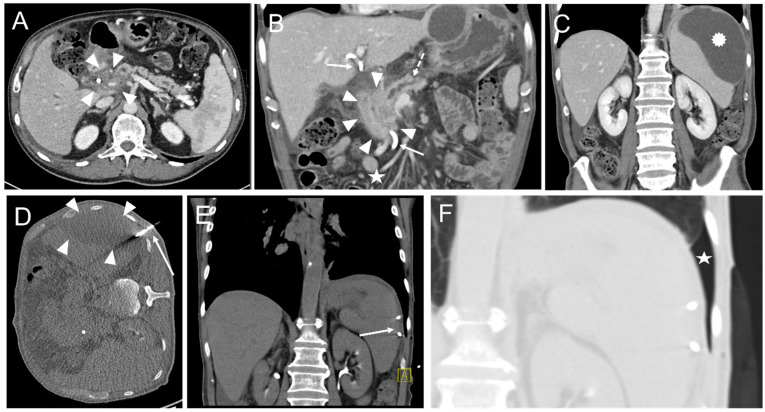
An intervention with a minor complication according to SIR. (A) 70-year-old male developing jaundice. CT scan depicts a pancreatic head tumor (arrowheads) with infiltration of the hepatic artery and spread along the portal vein as well as the mesenteric root. (B) Infiltration and congestion of the pancreatic duct (dashed arrow), enlarged and roundly configured lymph node metastases (asterisk), and a previously endoscopically inserted drainage (arrows). Arrowheads: pancreatic head tumor. (C) An exploratory laparotomy including multiple biopsy samples of the pancreas was conducted. The Patient developed postoperative pancreatitis. Twenty-seven days after surgery a pancreatogenic, perisplenic subcapsular pseudocyst (star) was diagnosed on a CT scan. (D) Placement of an 8F drainage (arrow) within the fluid collection (arrowheads) in the right lateral decubitus position, guided by CT fluoroscopy. (E) Postinterventional unenhanced control CT scan shows the correct position of the drainage (arrow) and an almost complete disappearance of the fluid collection. (F) However, a small self-limiting pneumothorax (star) was observed in the lung window.
